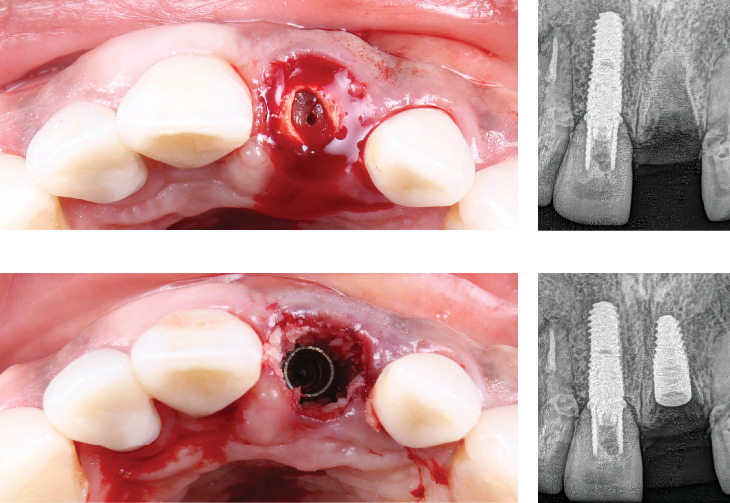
Extraction and implant placement. (a) Fractured tooth occlusal view, (b) radiograph of extracted tooth, (c) implant placement occlusal view, and (d) radiograph of implant placement.
